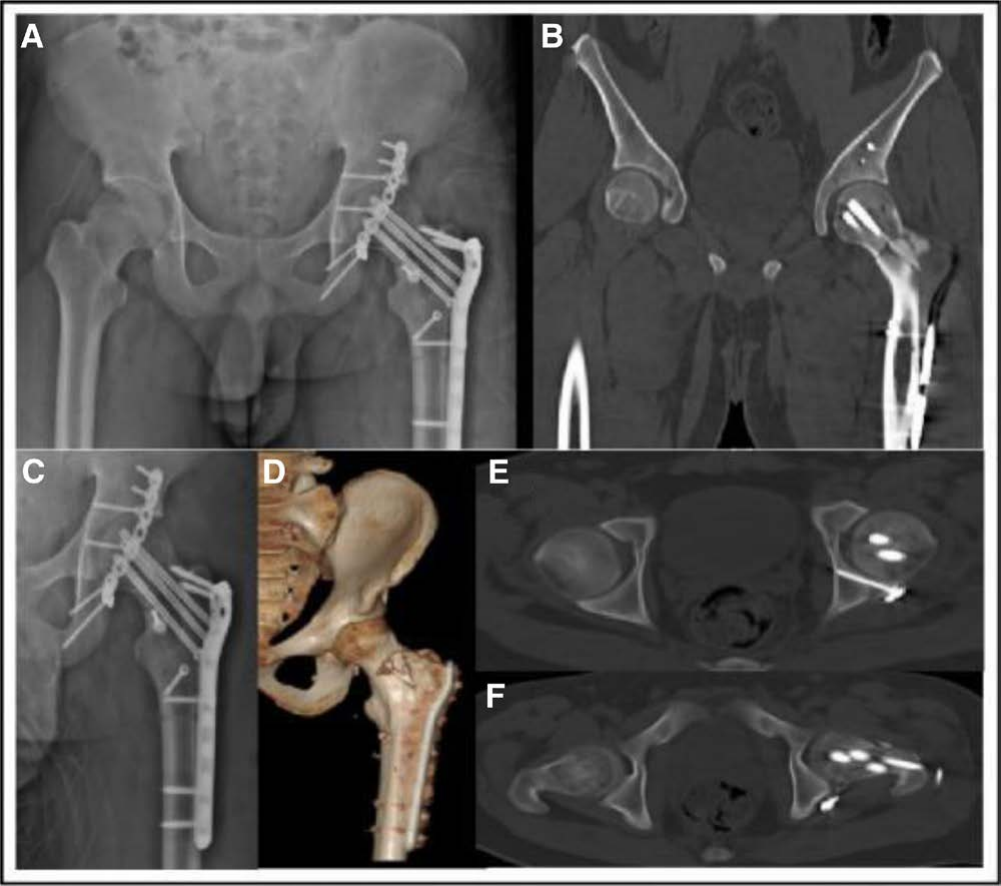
Postoperative DR and CT examination of the pelvis and hip showed that the fracture was well reduced, internal fixation was effective, the screw length was appropriate, and the femoral quadratus bone flap was in a satisfactory position. (B) Postoperative coronal CT images of the pelvis; (C, D) postoperative DR and CT 3D reconstruction of the hip joint; (E, F) postoperative transverse CT images of the pelvis. CT = computed tomography, DR = digital radiography.
